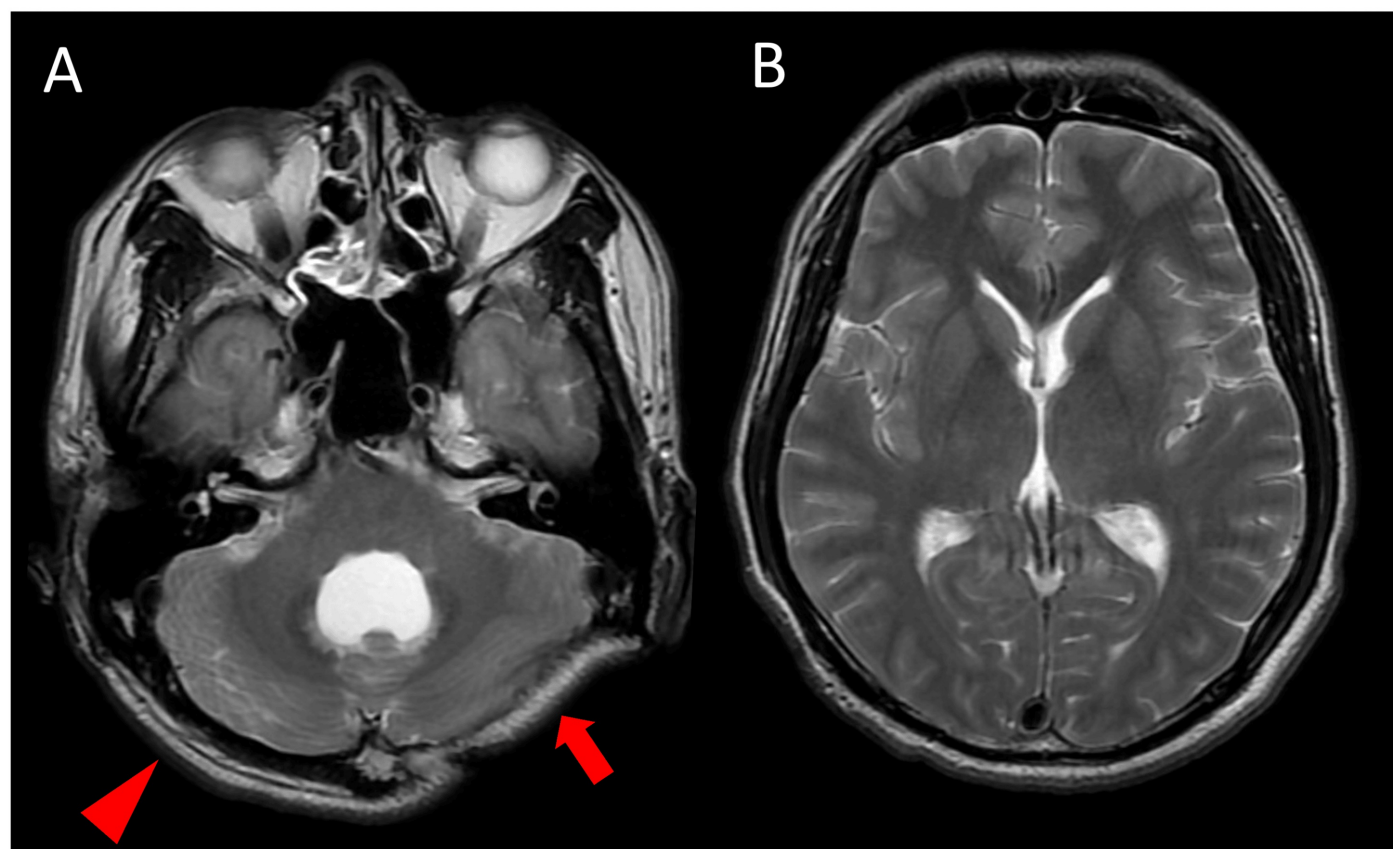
CT at readmission with orthostatic symptoms. (A) CT demonstrating enlargement of the fourth ventricle. Compared with the contralateral side (arrowhead), the skin flap on the affected side appears atrophic (arrow). (B) No enlargement of the lateral ventricles is observed.
